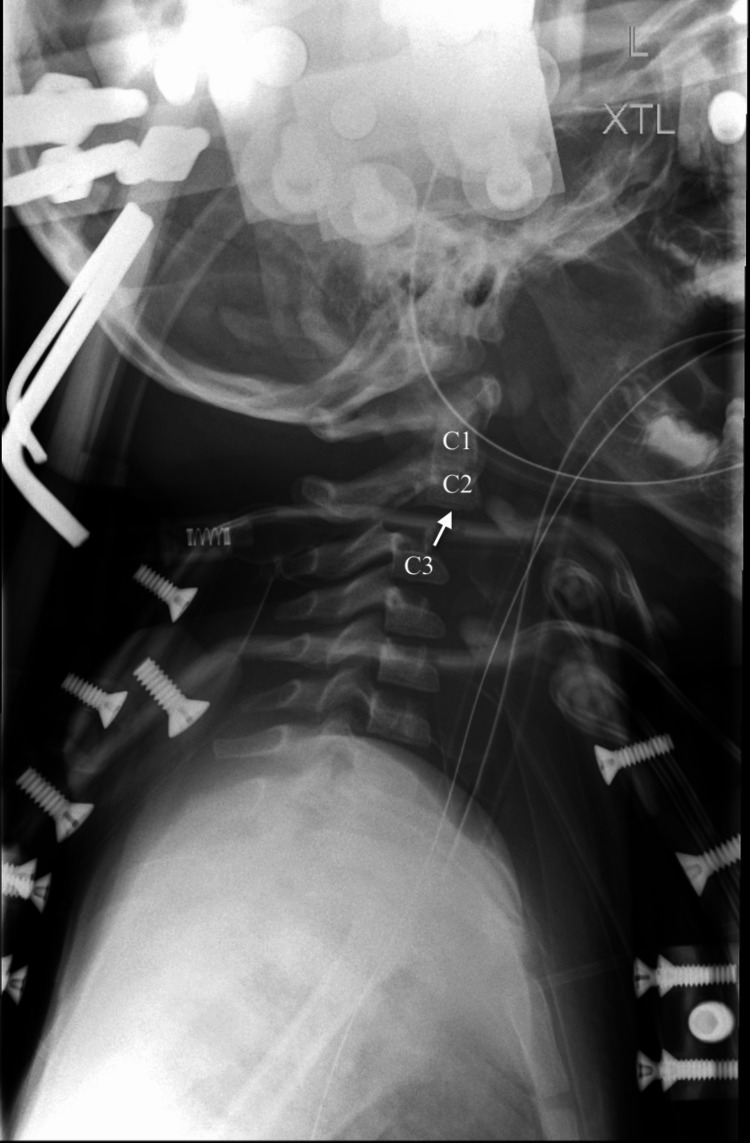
Lateral C-spine X-ray demonstrating unsatisfactory reduction with distraction and anterior subluxation of C2 relative to C3
